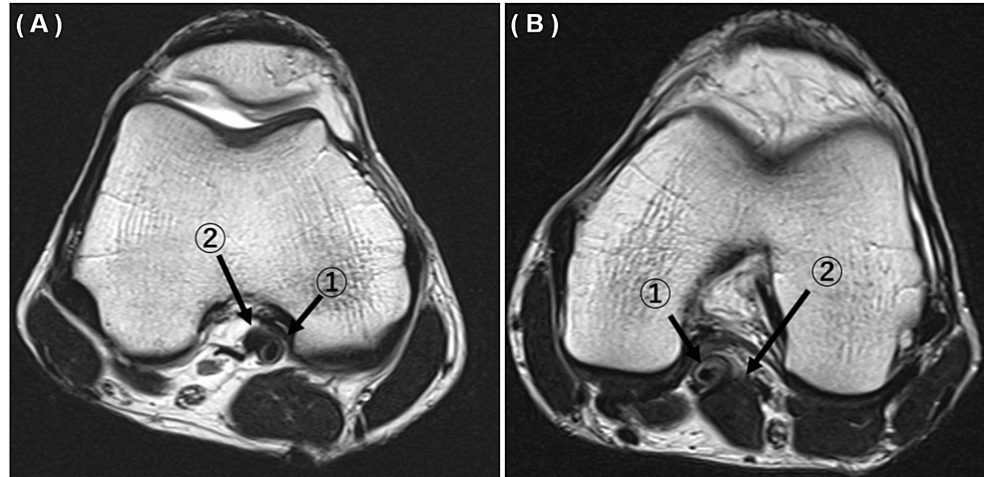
Magnetic resonance imaging of both legs. 1: Popliteal artery. 2: Medial head of the gastrocnemius muscle. A: Right knee. B: Left knee.
